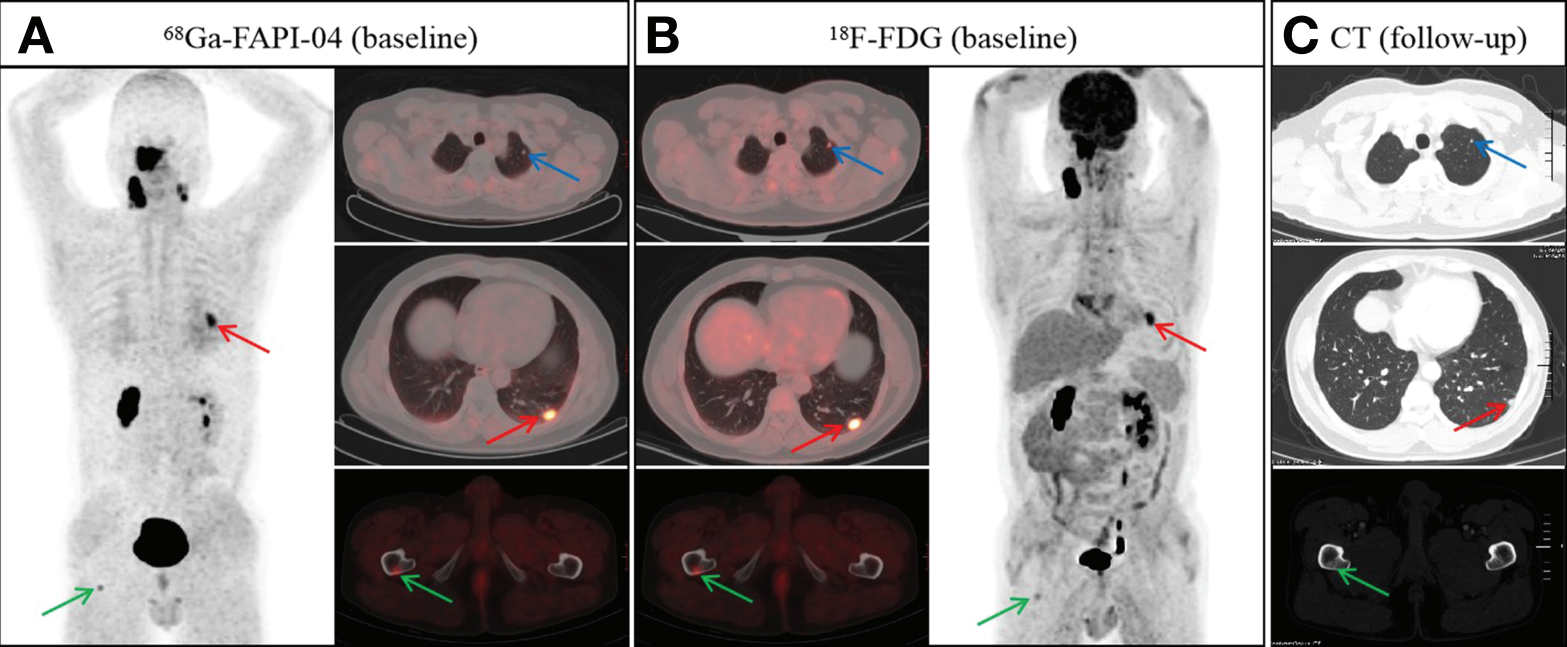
A 48-year-old man with nonkeratinizing undifferentiated carcinoma.^68^Ga-FAPI-04 (A) and ^18^F-FDG PET/CT (B) revealed an abnormal nodule in the left lower lobe (A, red arrow, SUVmax 4.9 vs. B, red arrow, SUVmax 8.2). However, the nodule in the left upper lobe showed abnormal uptake on ^18^F-FDG PET/CT (B, blue arrow), but not on ^68^Ga-FAPI-04 PET/CT (A, blue arrow). In addition, both ^68^Ga-FAPI-04 (A, green arrow) and ^18^F-FDG PET/CT (B, green arrow) revealed abnormal activity in the right femur. Follow-up CT after two cycles of induction chemotherapy showed a reduction in the volume of pulmonary metastases (C, red and blue arrow). Meanwhile, the bone metastasis of the right femur revealed a repair response after treatment, showing osteosclerotic nodule on follow-up CT (C, green arrow).
